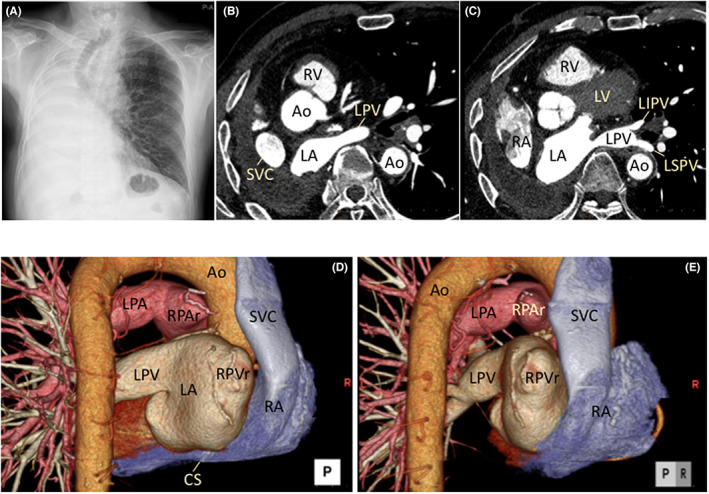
(A) Chest X‐ray showing the heart, bronchus, and esophagus, all displaced to the right after the lung cancer surgery. (B, C) Computed tomography scan revealing that the heart was deviated to the right. (D, E) Three‐dimensional reconstructed imaging on computed tomography showing the remnant of the right PV in contact with the SVC. The left PV had a common trunk. Ao, Aorta; CS, coronary sinus; LA, left atrium; LPA, left pulmonary artery; LI(S)PV, left inferior (superior) pulmonary vein; LPV, left pulmonary vein; RA, right atrium; RPAr, remnant of the right pulmonary artery; RPVr, remnant of the right pulmonary vein; SVC, superior vena cava.
